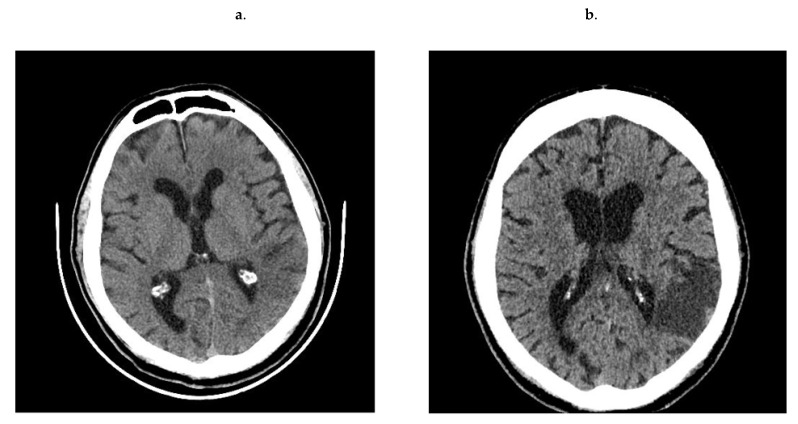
Ischemic lesion evolution between 1st day (a) and 7th day (b) in the no-contrast CT scans.
